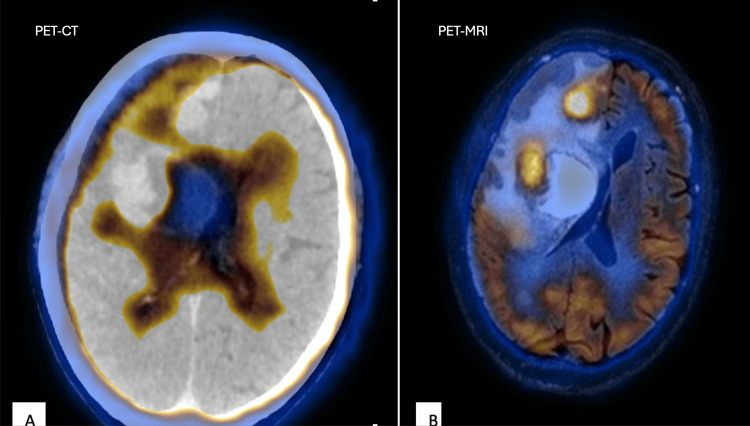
41-year-old female with a history of episodes of unconsciousness. (A) PET-CT shows an intense metabolically active ill-defined lesion in the right frontal region. (B) PET-MRI fusion was done to characterize the lesion. It shows a large solid cystic lesion in the right frontal region with surrounding edema and intense FDG uptake in the solid component, suggesting a primary neoplastic etiology. PET: positron emission tomography; MRI: magnetic resonance imaging; CT: computed tomography; FDG: fluorodeoxyglucose
